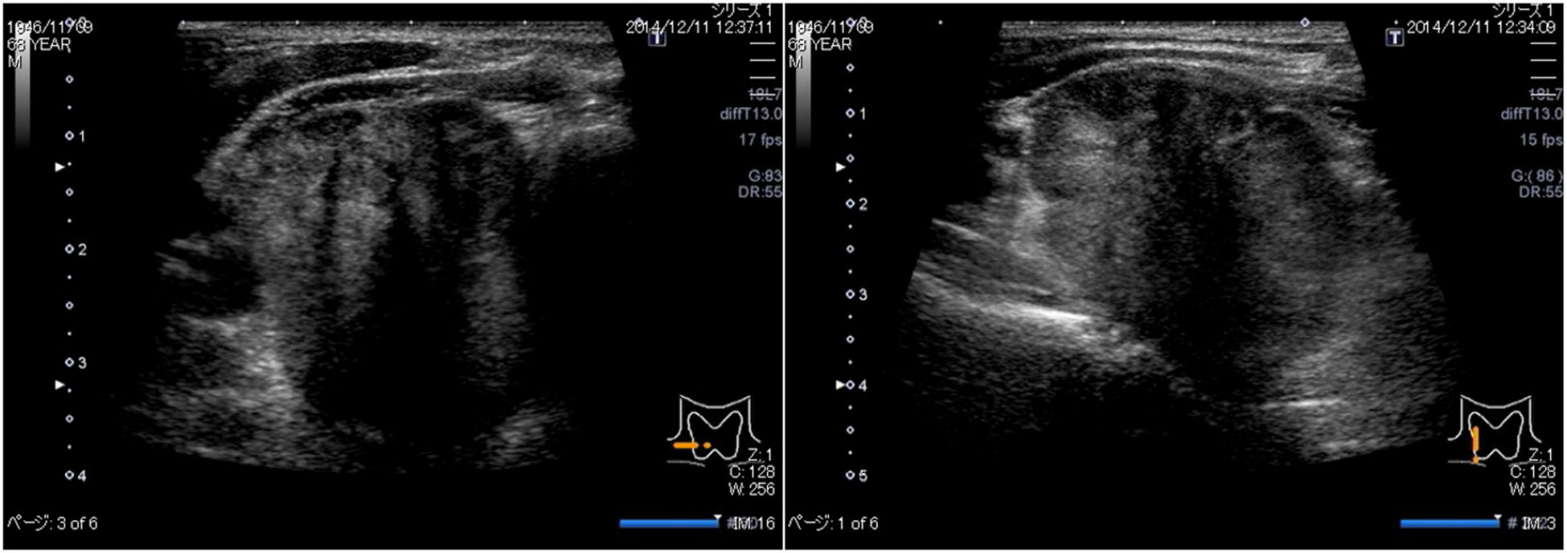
Ultrasound findings. A hypoechoic area of 4.7 × 3.4 cm with an irregular margin and heterogeneity was detected
